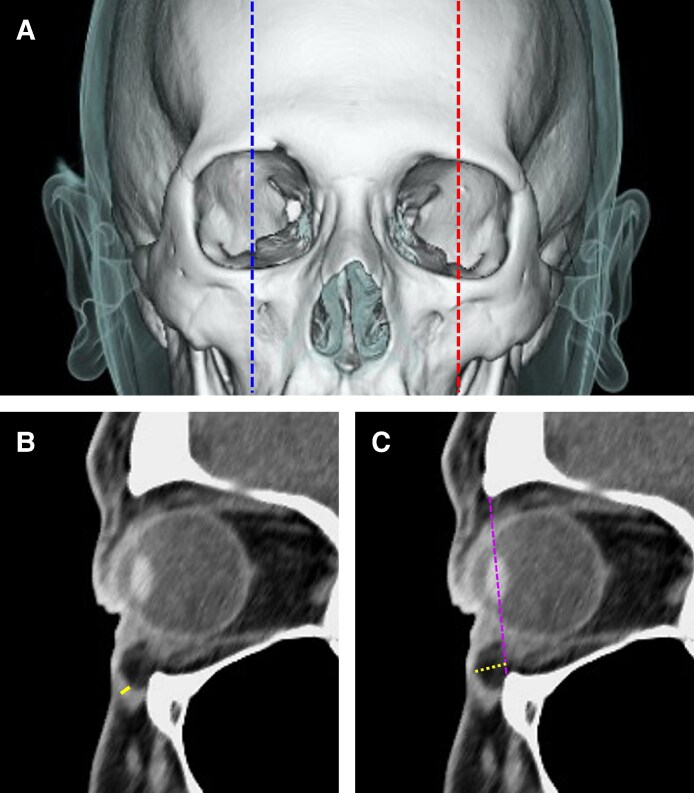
(A) Three-dimensional computed tomography (CT) image of the facial bone overlaid with a semi-transparent green skin layer. To assess orbicularis oculi muscle (OOM) thickness and the length of orbital fat herniation, sagittal CT images through the orbital center were reconstructed along the dashed lines for both the control (left: blue dashed lines) and neuromuscular electrical stimulation-applied (right: red dashed lines) sides. (B) Reconstructed sagittal CT image. OOM thickness is defined as the distance between the anterior and posterior surfaces of the muscle, indicated by the yellow line. (C) Another reconstructed sagittal CT image. The purple dashed lines running from the anterior edge of the orbital roof to the anterior edge of the orbital floor was used as a reference for measuring orbital fat prolapse length. Orbital fat prolapse length is defined as the length of fat herniation (measured as the length of the straight orange line).
